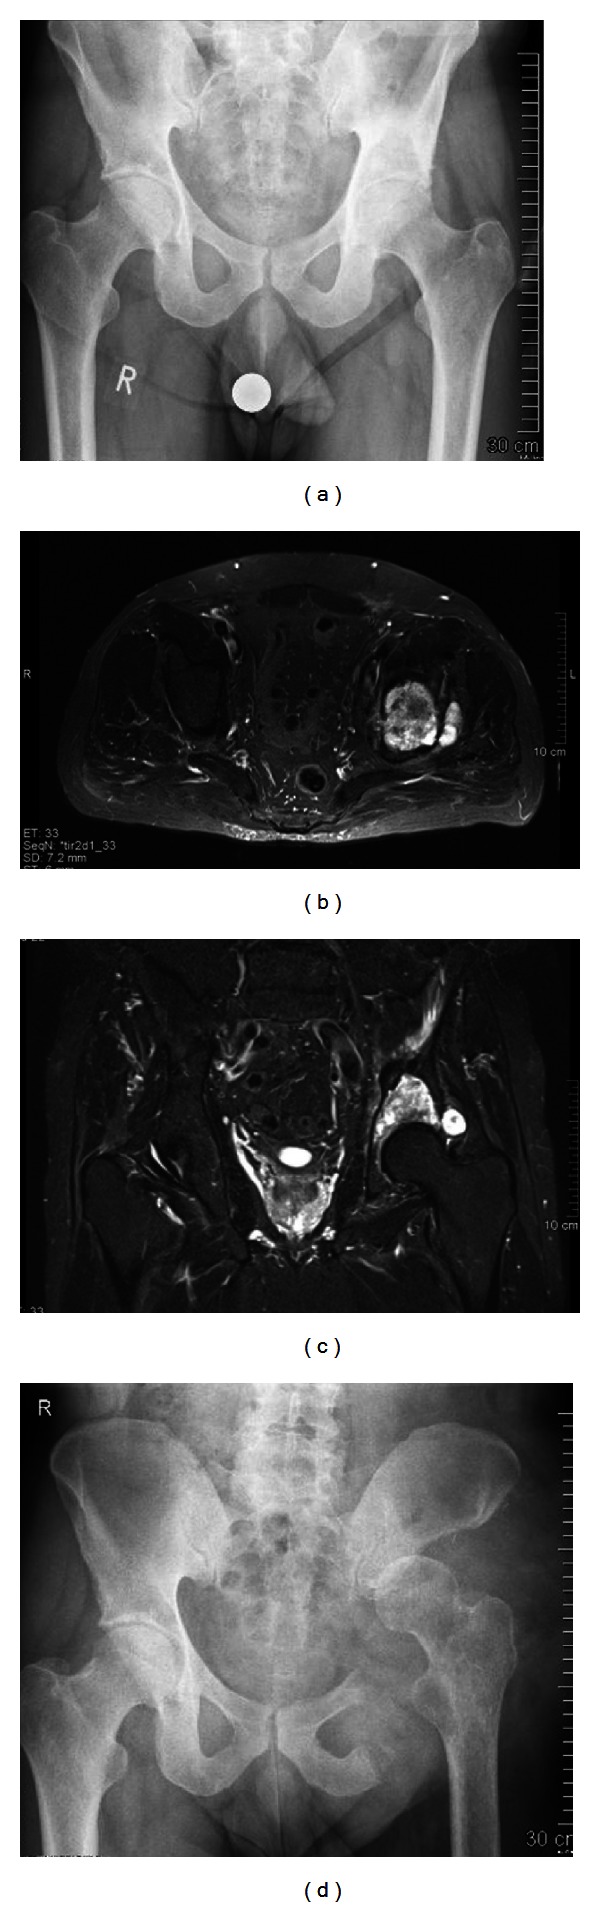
(a) Anteroposterior radiograph of the pelvis, showing a periacetabular chondrosarcoma on the left. (b) and (c) MRI of the pelvis, showing the destruction of the cortical bone and extraosseous tumor expansion. Notably is that the hip joint is not infiltrated. (d) Anteroposterior radiograph after P2 resection and hip transposition.
